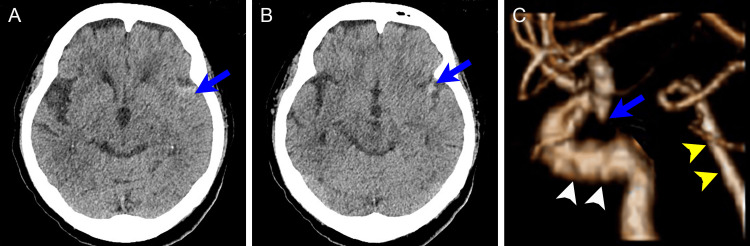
Head CT scan obtained at the symptom onset (A, B) Plain head CT scan shows high-density deposits in the left Sylvian fissure (arrows). Sulcal effacement of the left cerebral hemisphere was observed. (C) A lateral view of the 3D-reconstructed CT angiogram shows a small left posterior communicating artery aneurysm (arrow). White and yellow arrowheads indicate the internal carotid artery and the basilar artery, respectively.
